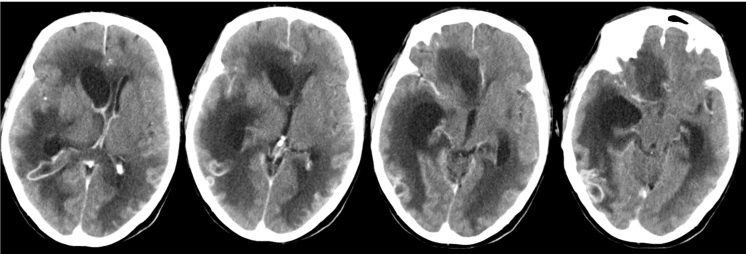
Contrast-enhanced CT scan: Intracerebral lesions involving the deep callus and white matter, especially on the right side. There is a diffuse sulcal leptomeningeal enhancement and on the ventricular contour and multiple lesions with temporo-occipital nodular enhancement. These aspects reflect the presence of brain abscesses and meningitis aggravated with ventriculitis and hydrocephalus.
